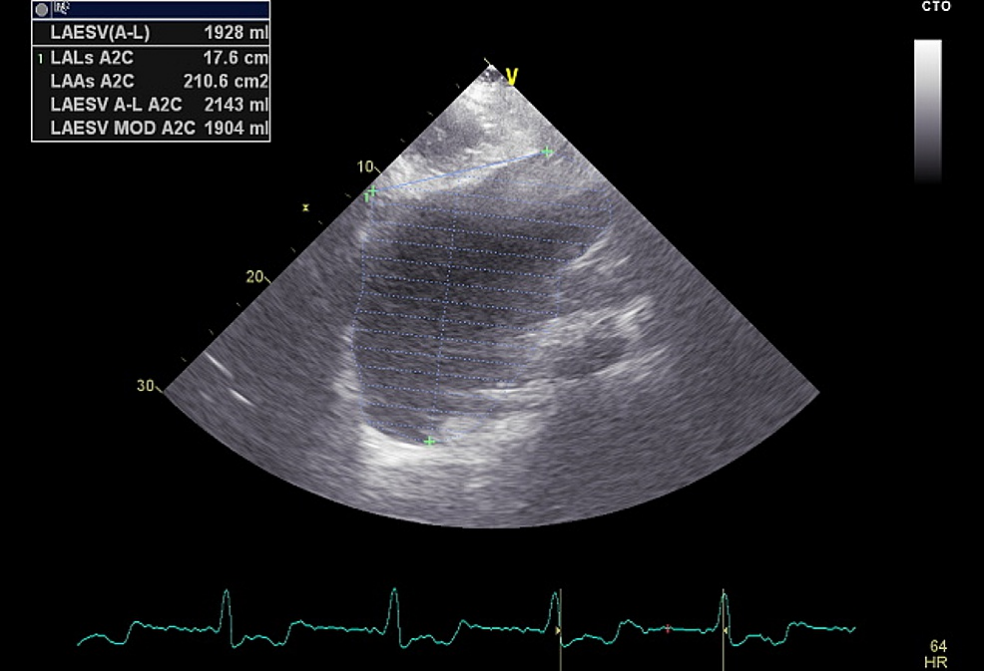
Echocardiography image of the left atrium (LA area)
